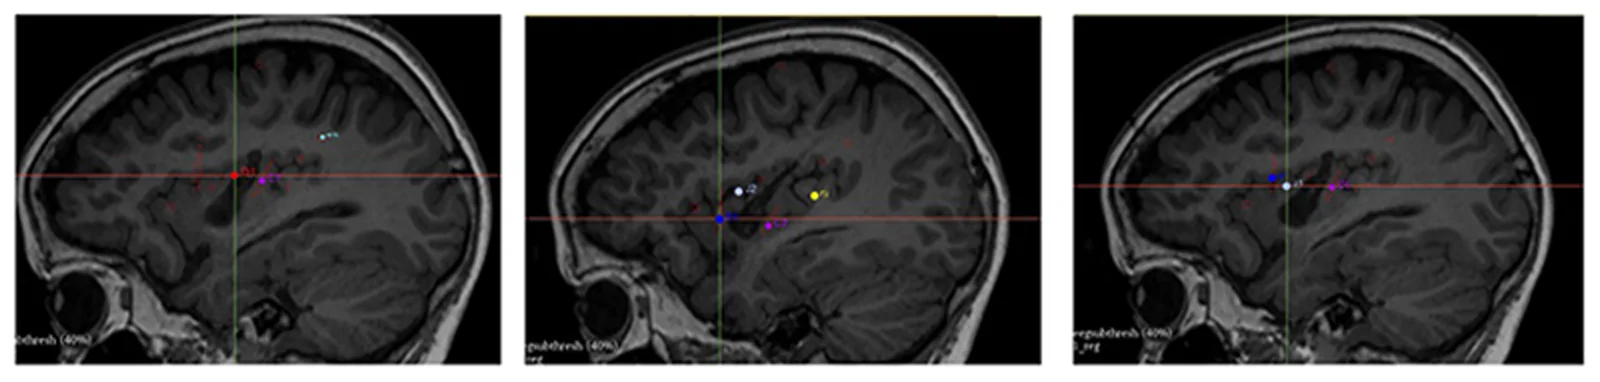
Sagittal T1-weighted volumetric MRI slices demonstrating the location of the 3 highlighted involved contacts on electrodes D (left, red), B (middle, dark blue) and J (right, light blue) shown in Fig. 3, all anterior to the area of laser ablation just posterior to the highlighted contact D1 (red). Crosshair highlights the location of the contact highlighted in Fig. 4 showing fast activity.
